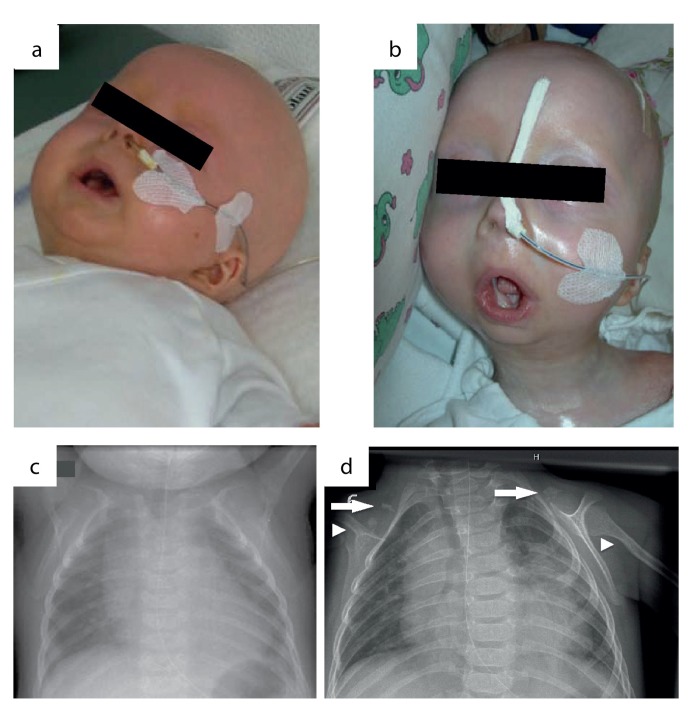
Phenotypic changes in the course of disease progression at age of 4 months (a) and age of 11 months (b). Chest x-rays were performed at age of 4 (c) and 11 months (d). Normal skeletal findings at age of 4 months are displayed. Missing of both claviculae at age of 11 months, residual bone fragments are denoted by arrows. Deformation and narrowing of both humeri at age of 11 months are marked by arrowheads.
